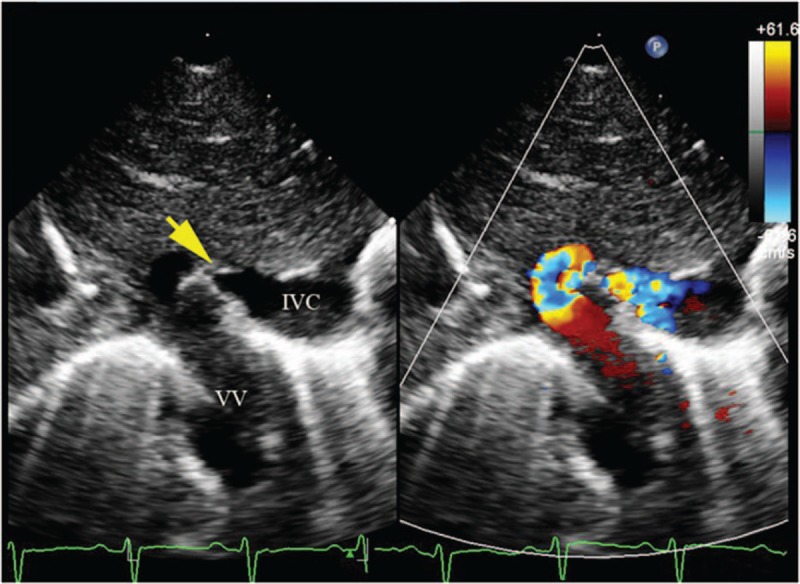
A 6-day-old baby with infracardiac anomalous pulmonary venous connection with descending vertical vein (DVV) obstruction. (A) Two-dimensional echocardiography showing the obstruction at the site of the DVV draining into the inferior vena cava, color Doppler shows the turbulence flow at this site. (B) Pulse Doppler of flow in the anomalous pulmonary vein with obstruction shown as nonphasic with high velocity.
